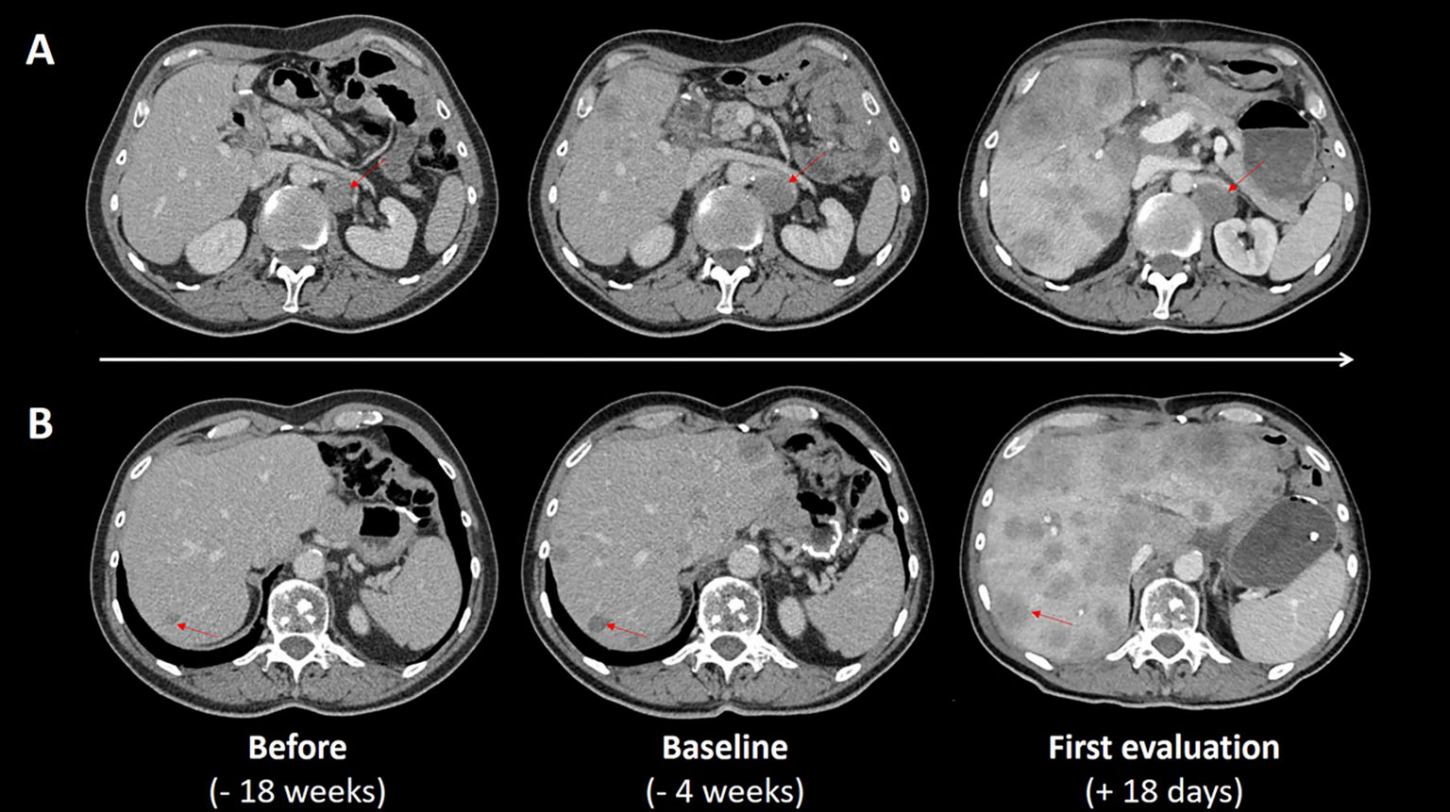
CT scan evaluations before and after treatment. CT scans performed 18 weeks before ICI treatment (first column), at baseline (second column) and for early evaluation eighteen days after the beginning of ICI treatment (third column) show the changes in lymph nodes and hepatic lesions: the left para-aortic lymph node (red arrow, line A) increased from 30mm at baseline to 40mm on day 18, known hepatic lesion increased (red arrow, line B), multiple new hepatic lesions appeared and locoregional invasion led to gastric stump compression.
